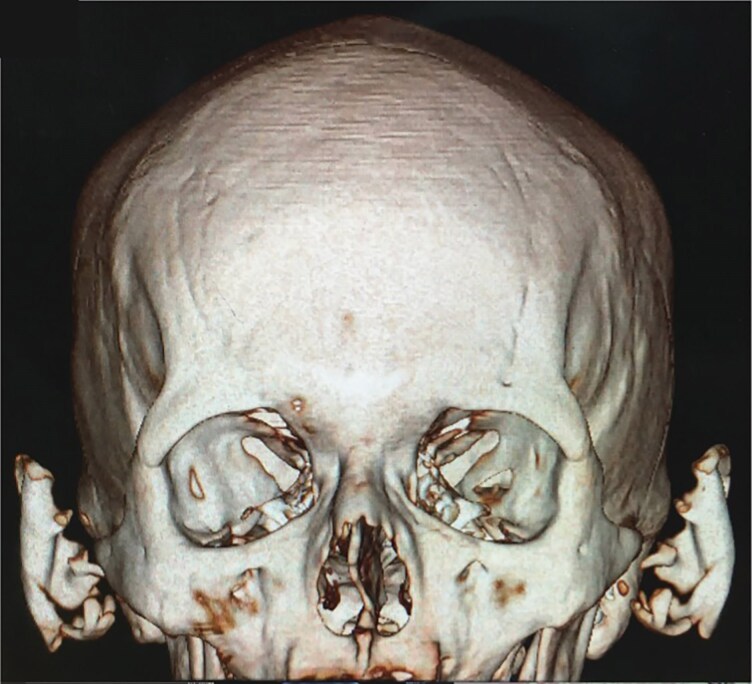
Computed tomography three dimensional reconstruction showing complete calcification of auricular cartilage in both ears.
